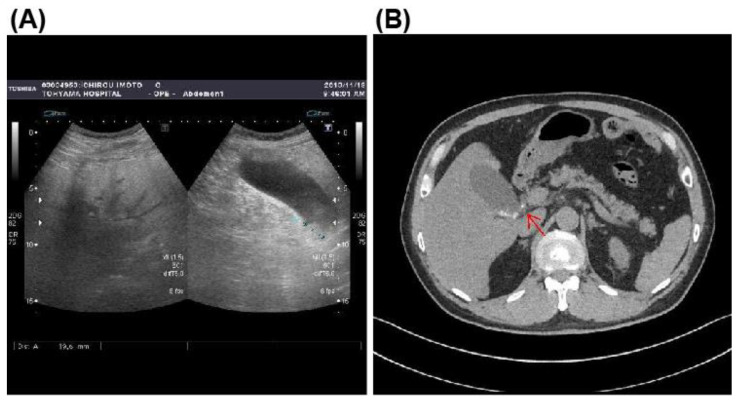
Abdominal ultrasonography and computed tomography findings. (A), Abdominal ultrasonography revealed moderately fatty liver and numerous small stones and sludge in the gallbladder. (B), Abdominal CT revealed small stones in the enlarged gallbladder with suspicion of stone impaction (arrow) in the gallbladder neck.
